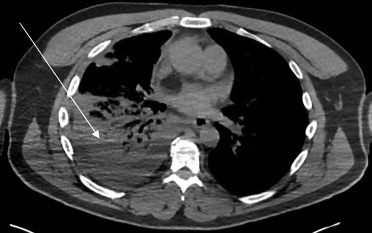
Non-contrast enhanced computed tomography of the chest. Arrow pointing at multilobular atelectasis and pleural effusion.
